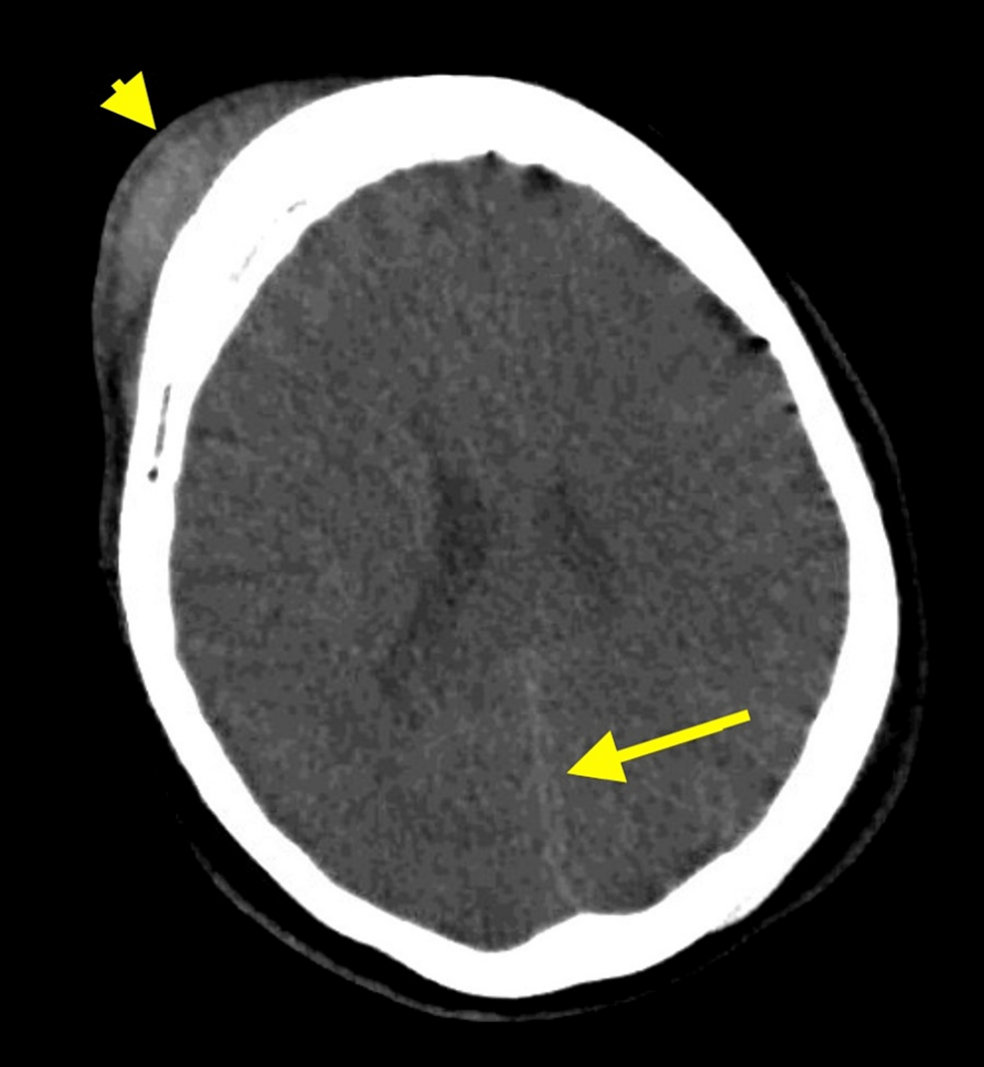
CT head without contrast on admission. An axial view of CT of the head without contrast demonstrates a large right frontal scalp hematoma (arrowhead) and 3 mm interhemispheric subdural hematoma (arrow) without midline shift. CT, computed tomography
